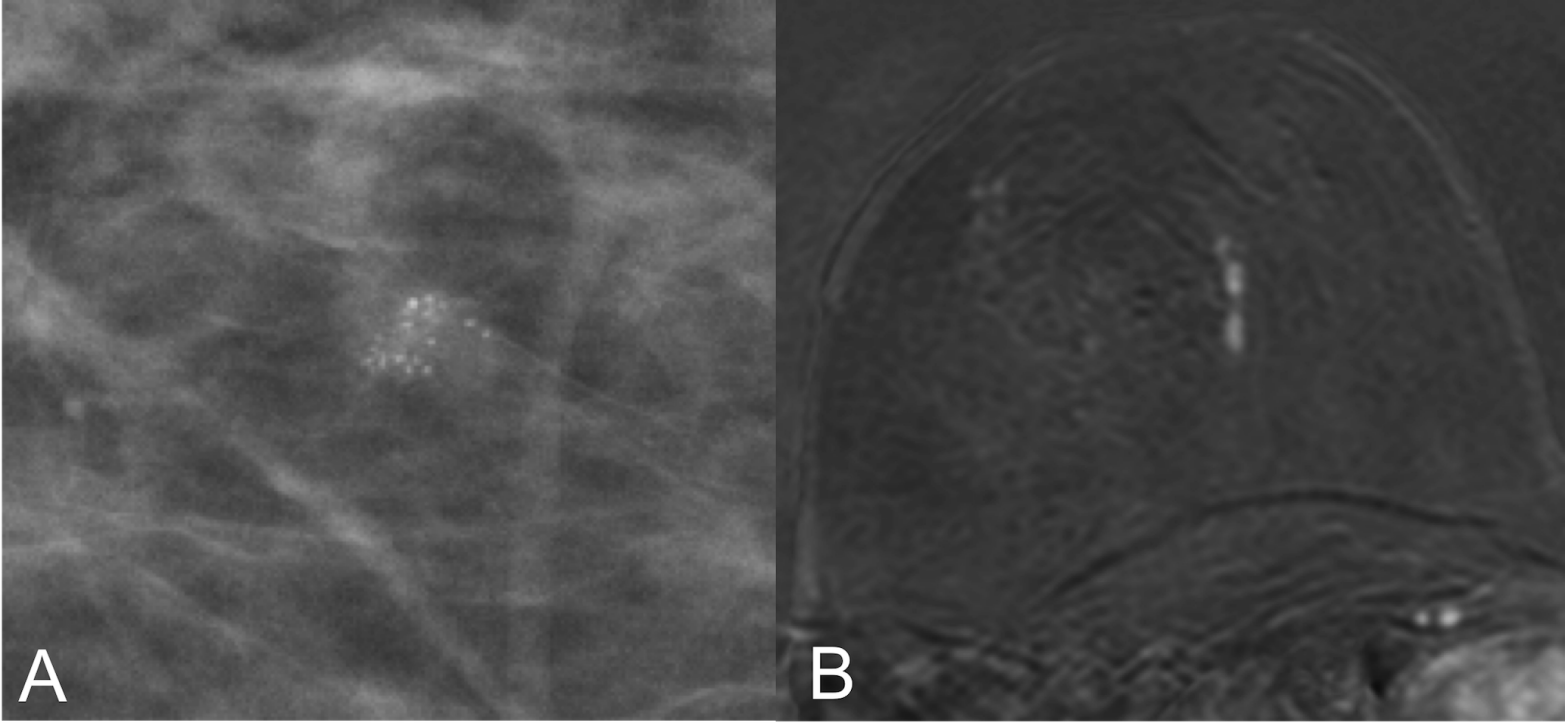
Small cluster of screening detected BI-RADS 4b microcalcifications (A) in the right breast of a 50-year old woman. MRI (B) demonstrates a linear clumped non-mass enhancement rated BI-RADS 4. Vacuum-assisted breast biopsy and subsequent surgery revealed a hormonal receptor positive DCIS G2.
